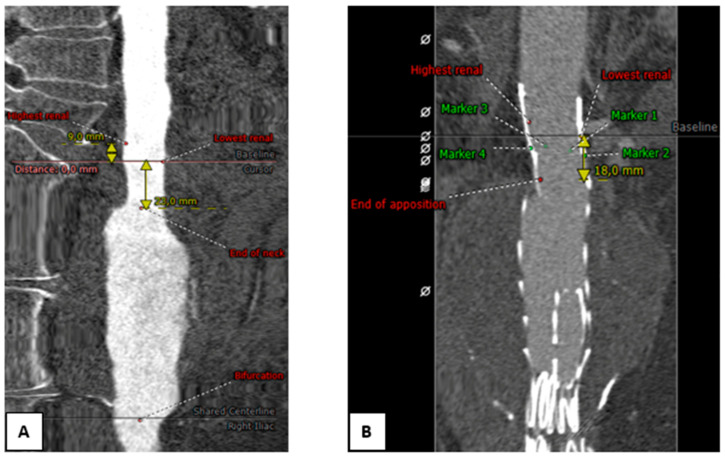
Measurements in 3mensio vascular workstation on the preoperative computed tomography angiography (CTA) scan. (A) Baseline is set to the distal edge of the lowest renal artery. The end of the aortic neck is defined as the first slice that exceeds a 10% diameter increase compared with baseline; (B) positioning of three-dimensional coordinate markers. CTA coordinates are obtained from the lowest and highest renal arteries (red), the end of circumferential apposition (red), and of four radiopaque markers that define the proximal edge of the endograft fabric (green).
